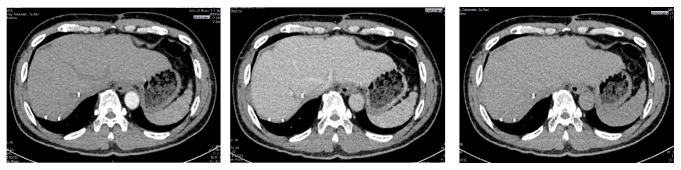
Arterial, venous, and delayed phase of Abdominal CT Scan 4 months after surgical resection (February 2014).
